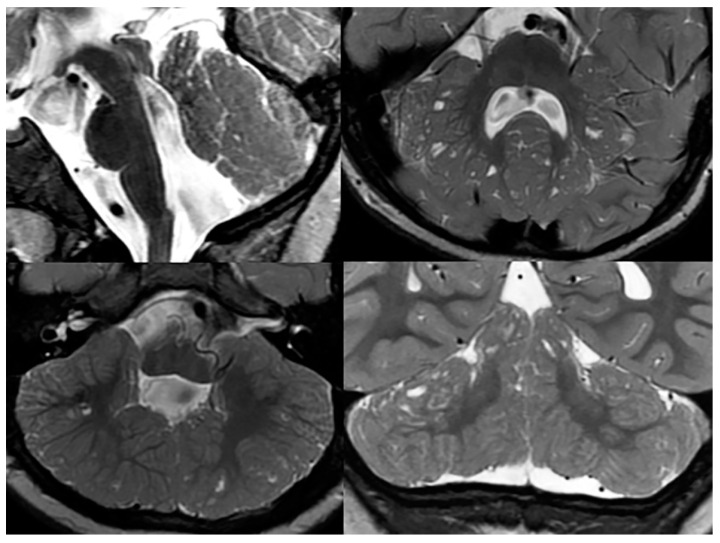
Sagittal, and axial and coronal T2-weighted MRIs of a patient with confirmed Poretti-Boltshauser syndrome show the characteristic elongation of the mesencephalon, disorganized cerebellar foliae, and multiple cerebellar inclusion cysts.
